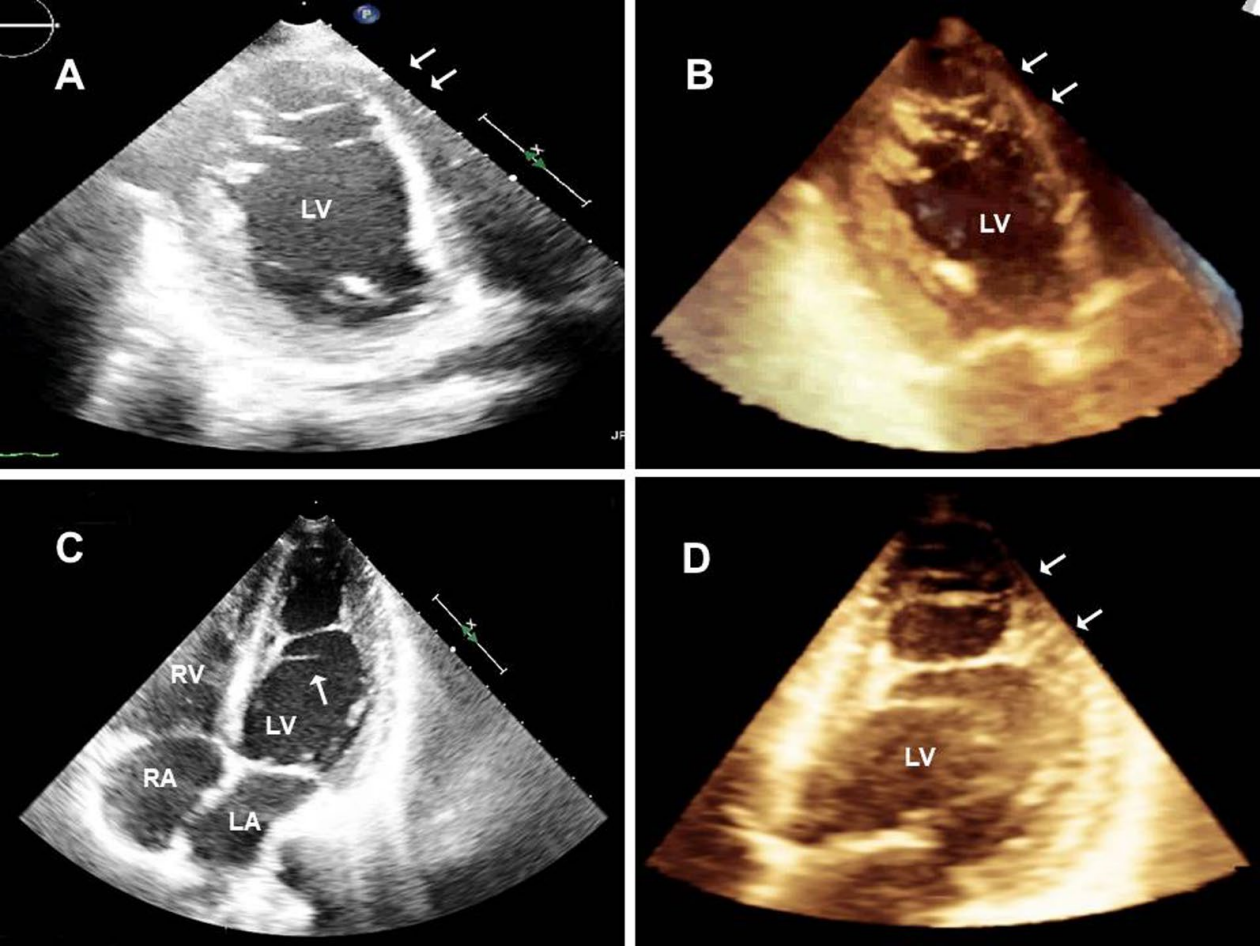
A, C. Modified two-dimensional echocardiographic examination of two patients shows only two left ventricular false tendons (arrows). However, B, D three-dimensional examination of the same images reveals multiple, mesh-shaped, complex left ventricular false tendon structures (arrows)
